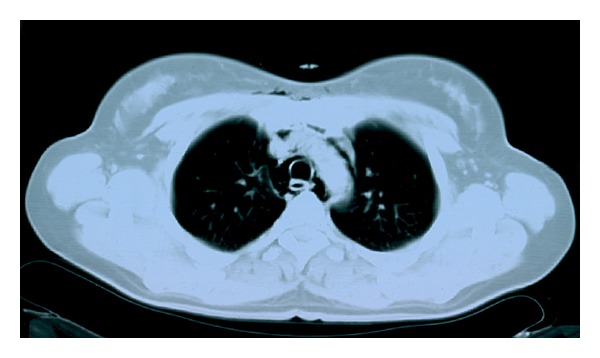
Axial computed tomography of the chest at the level of the aortic arch reveals the presence of air in the mediastinum.
